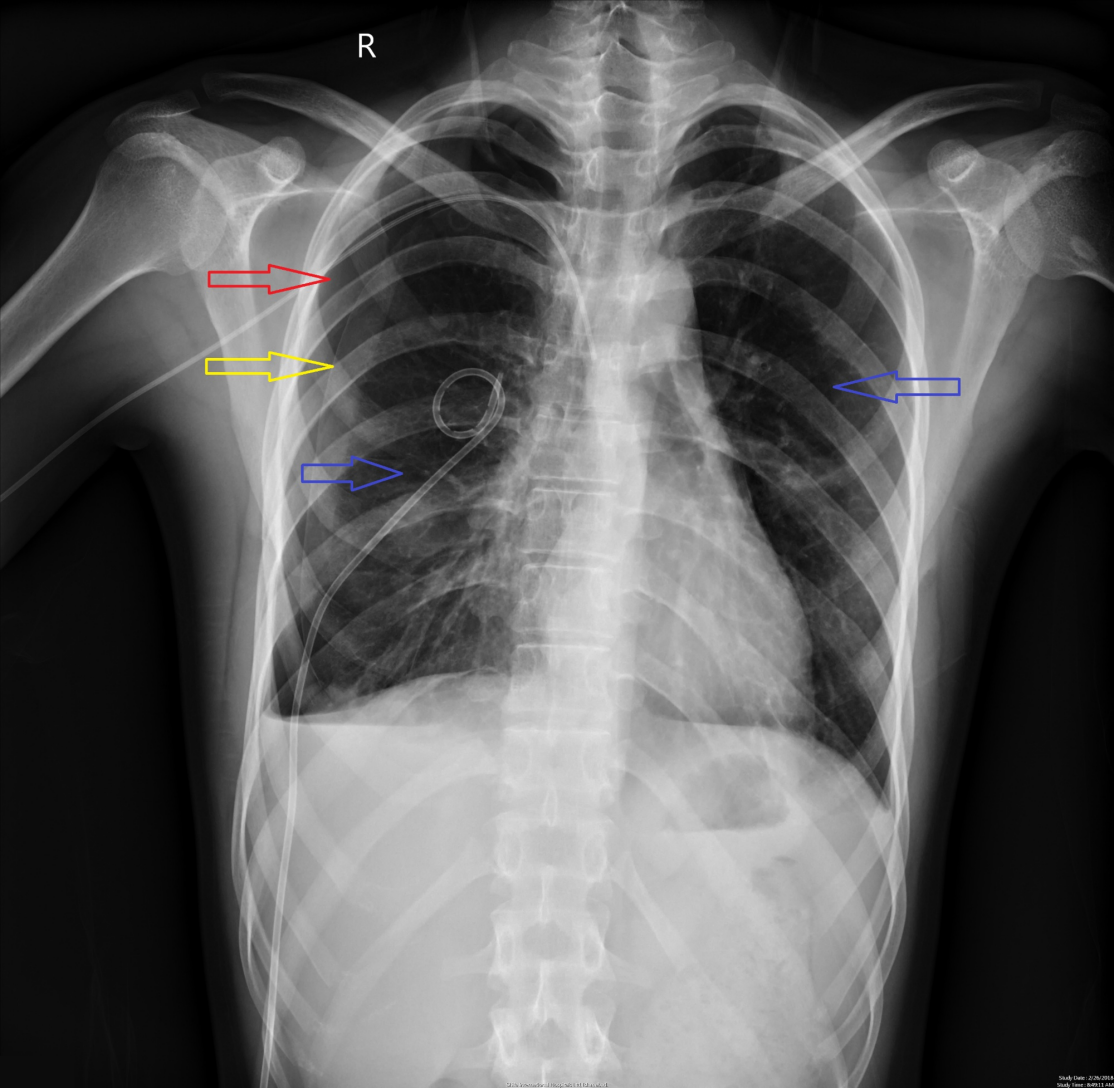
Chest X-ray showing resolving pneumothorax bilaterally. Red arrow points toward an area of radiolucency in the right thorax indicating a partially resolved pneumothorax. Yellow arrow points toward the beginning of the lung parenchyma on the right side indicating a partial pneumothorax on the right side. Blue arrows point toward normal lung parenchyma.
